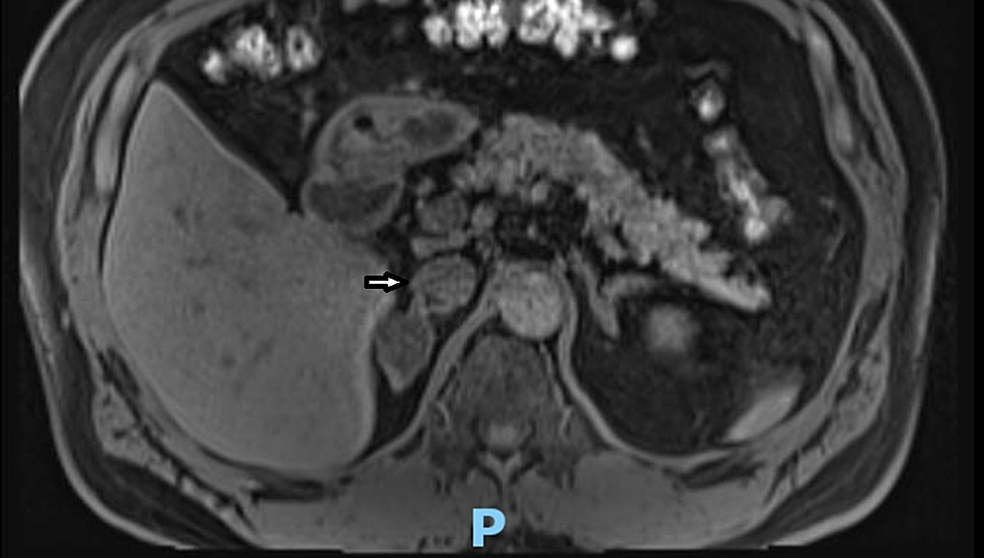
MRI adrenal glands There is a 2.4 x 2.0 cm ovoid nodule arising from the right adrenal gland lateral limb (pointed by an arrow). The left adrenal gland appears normal.
